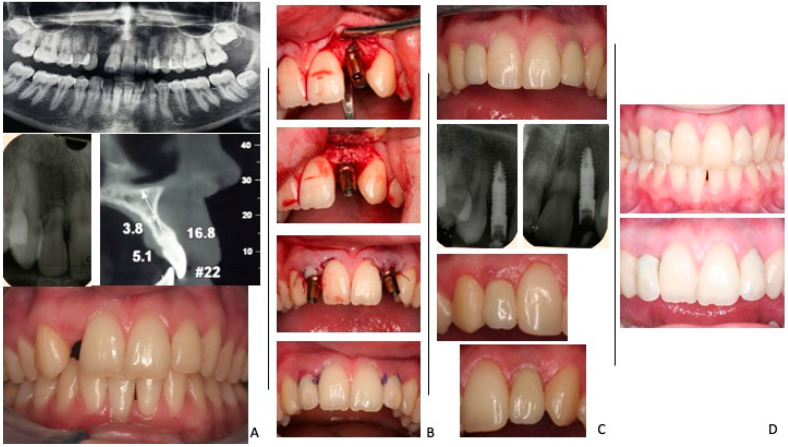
(A) Preoperative radiographic and clinical presentation of a patient with failing tooth #22 indicated for extraction and implant placement, and a congenitally missing #12, (B) Intraoperative view of immediate implant placement at the site of tooth #22 extraction, with simultaneous bone augmentation. Delayed implant placement at the site of tooth #12 extraction. (C) Final implant-supported restorations in place on both #12 and #22. (D) Ten-year follow-up clinical photograph demonstrating healthy peri-implant tissues.
